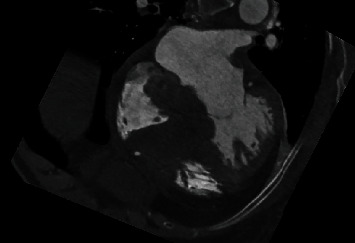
Four chamber view from the cardiac computed tomography angiogram demonstrating the mass invading the interatrial septum and inlet ventricular septum, and extending into the tricuspid annulus as well.
